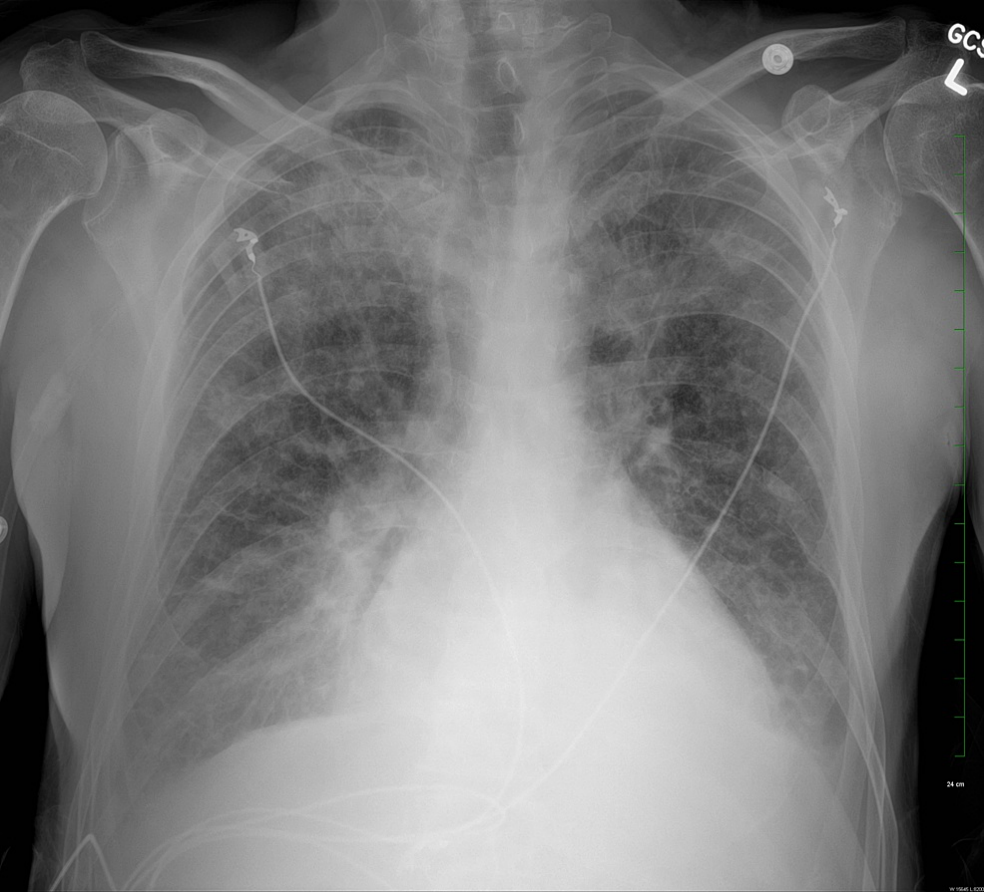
Chest X-ray revealing mild exacerbation of airspace opacities and bilateral pleural effusion during the seventh day of hospitalization.
